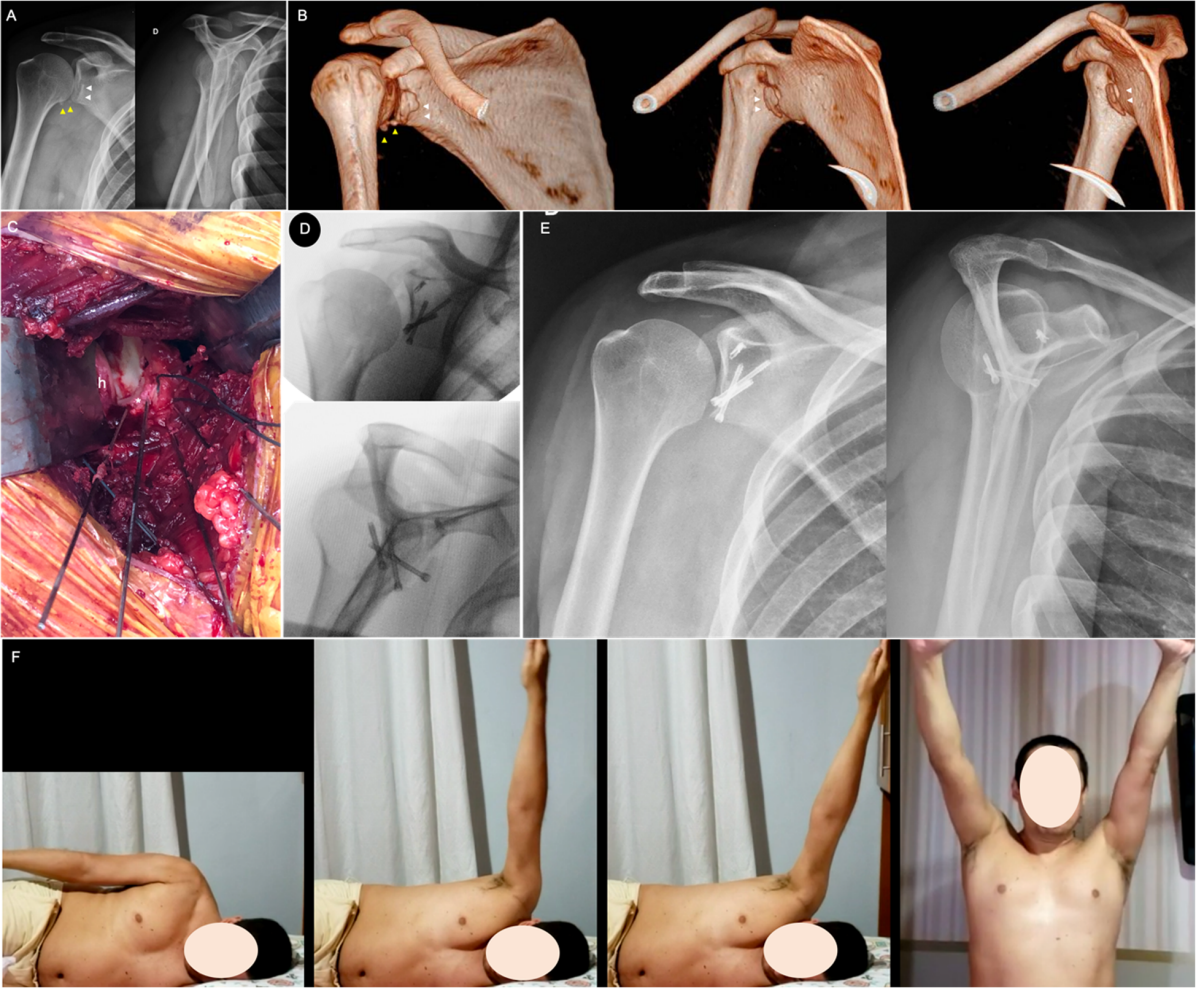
a: Preoperative true AP and lateral scapular radiographic views of the right shoulder of a 40-years-old male patient, showing a step-off on the anteroinferior rim of the glenoid (white arrowheads). Patient reported on a fall from stairs 48 h before. Also note the small bone fragments in the inferior portion of the capsule (yellow arrowheads); b: Preoperative 3-D CT reconstructions showing the displaced anteroinferior glenoid rim fracture (white arrowheads) and small bone fragments in the inferior portion of the capsule (yellow arrowheads); c: Intraoperative image showing the anteroinferior rim fracture anatomically reduced and provisionally fixed with multiple threaded K-wires. Observe the number 2 ethibond® sutures attached to the anterior labrum for posterior repair. * – anteroinferior glenoid rim fragment, h – humerus head; d: Intraoperative true AP and lateral scapular fluoroscopic views of the right shoulder showing final fixation with three 2.4-mm headless screws. Labrum was repaired using a bone anchor and unabsorbable sutures; e: Postoperative true anteroposterior and lateral scapular radiographic views of the right shoulder demonstrating the anatomic reduction of the anterior glenoid rim; f Pictures done during the rehabilitation protocol, demonstrating a satisfactory range of motion of the operated shoulder
